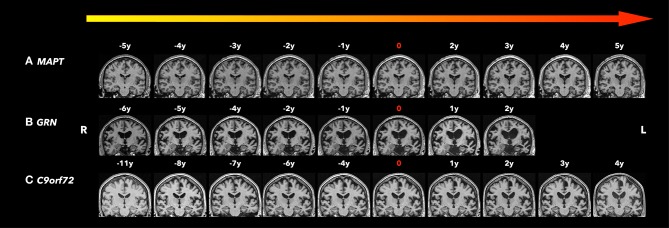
Coronal T1-weighted magnetic resonance imaging (MRI). Brain MRIs (coronal T1-weighted images) of the three familiar FTLD mutation carriers who progressed from the asymptomatic to the symptomatic stage are shown in the timeline with years before and after conversion. 0 indicates the actual symptom onset time point. (A) A male patient with *MAPT* N279K mutation whose first clinical manifestation was difficulties in remembering street names and people's names with Kokmen short test of mental status (36/38) at age 43. Two years after symptom onset, his forgetfulness progressed, with resting tremor in the left lower limbs, as well as some impulsivity and disinhibition, and was diagnosed with bvFTD and Parkinsonism. Five years after symptom onset, his cognitive function, parkinsonism, and behavior changes significantly worsened and mostly by motor impairment. Focal adjacent mesial temporal lobe atrophy mainly in the right side was observed 5 years before symptom onset and became bilateral hippocampal atrophy with generalized cerebral atrophy most marked in the bilateral parietotemporal regions. The structural brain change seems to be slower than his clinic symptom progression. (B) A male patient *GRN* mutation carrier whose first clinical manifestation was word-finding difficulties at age 68. Two years after symptom onset, his word-finding difficulties progressed, with additional executive dysfunction, forgetfulness, and tendency to repeat questions. He also had apathy, irritability, and dysphoria, and was diagnosed with mixed PPA/bvFTD syndrome. Asymmetrical (left greater than right) atrophy was observed 6 years before symptom onset, and became progressively asymmetric affecting the frontal, temporal, and parietal lobes as he aged. (C) A male patient *C9orf72* mutation carrier whose first clinical manifestation was a change in motivation, spontaneity, and empathy at age 73 and was diagnosed as behavior MCI. After 4 years from symptom onset, his behavior change slightly progressed with subtleties in empathy, emotional blunting, irritability, and subtle apathy, still diagnosed as behavior MCI. While his clinic symptoms seem to be stable, the moderate parenchymal volume loss was observed 8 years before symptom onset most involved in the frontal and temporal areas, and progressed affecting the whole brain with greatest atrophy in the fronto-temporal regions as he aged.
